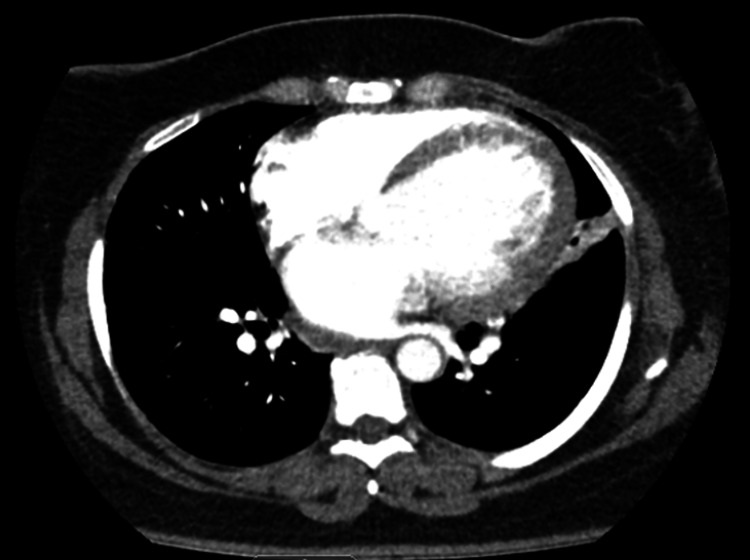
Chest CT scan (axial view) This view shows ground glass opacities on top of the heart enlargement.
